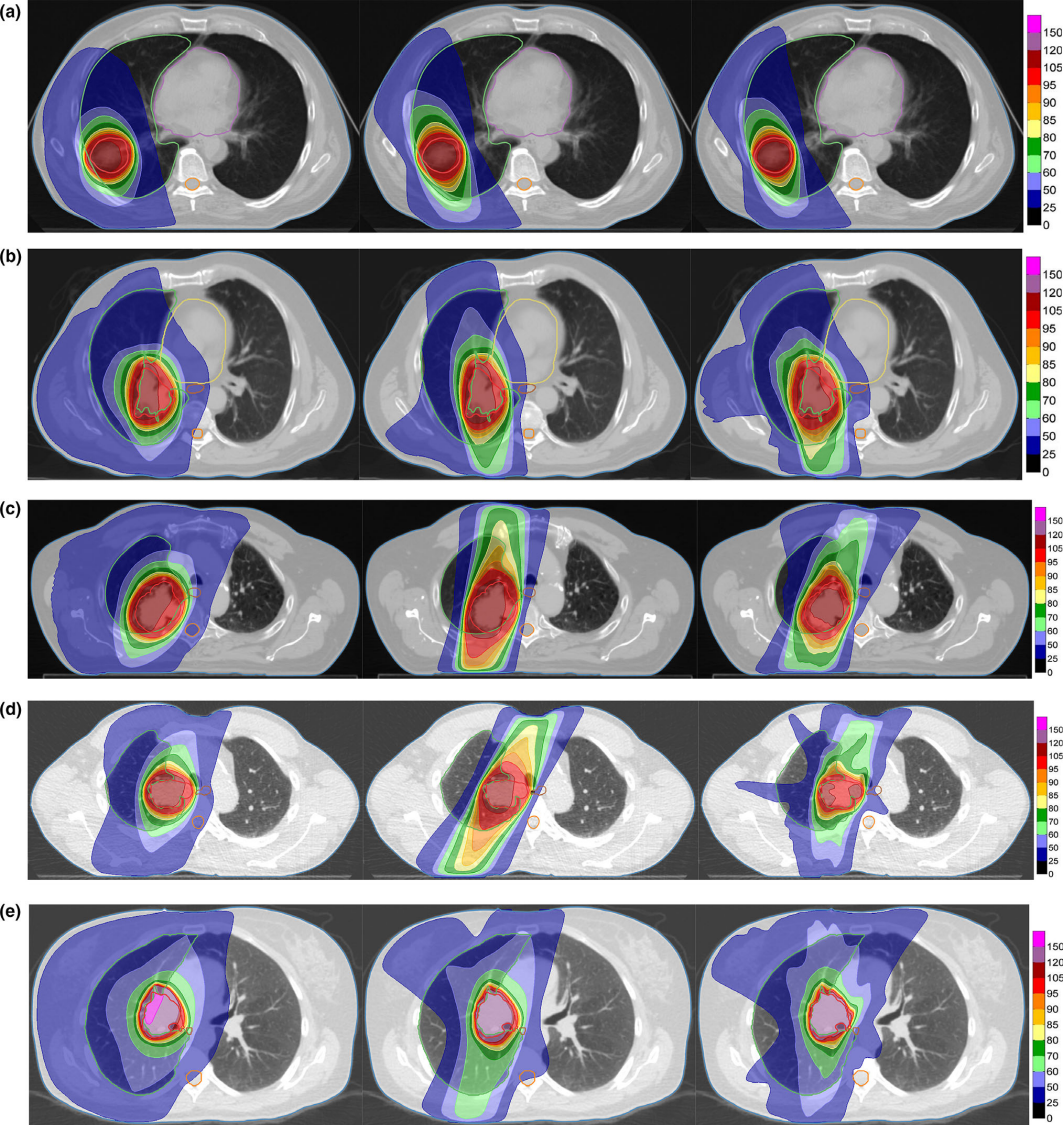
Dose distributions for the five patient cases for a transversal cut through the isocenter, overlaid on the planning computed tomography (CT). The plans shown in each subfigure are (from left to right): dynamic conformal arc (DCA), segment‐weight optimized (SWO)‐DCA, and volumetric‐modulated arc therapy (VMAT). Contours for regions of interests (ROIs) are indicated by solid lines according to the color scheme of Fig. 2. The color table is in percent of the prescription dose (60 Gy). (a) Patient 1, (b) Patient 2, (c) Patient 3, (d) Patient 4, (e) Patient 5.
